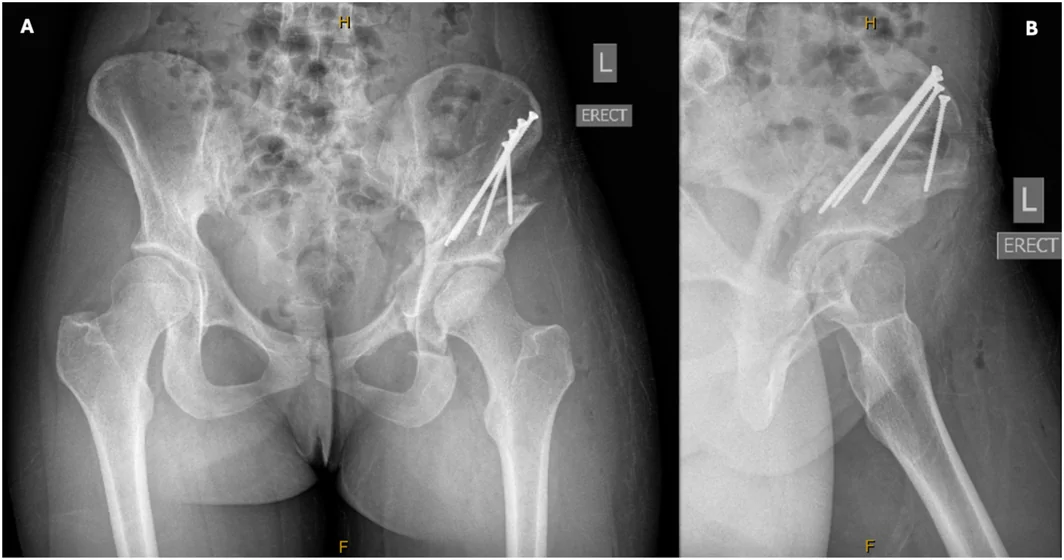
Postoperative radiographs demonstrating the achieved correction and appropriate screw placement following PAO in AP (A) and iliac oblique (B) views.
